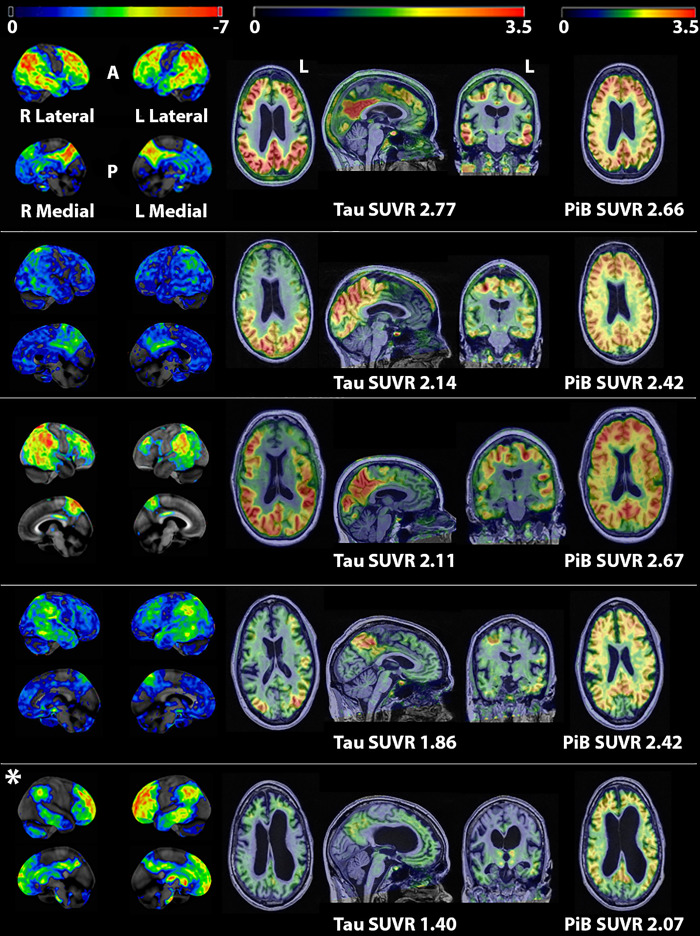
Discordance of CSF p-tau biomarkers and tau PET in five participants who had CSF p-tau in the normal range (< 61 pg/ml). *Left:* FDG–PET (using Cortex ID, GE Healthcare) with z-score bar at the top. *Middle:*^18^F-AV1451 tau PET with SUVR bar at the top and individual meta-ROI SUVR below each image. *Right:* PiB amyloid PET with SUVR bar at the top and individual meta-ROI SUVR below each image. Significant ^18^F-AV1451 uptake was present in all five participants (mean SUVR 2.05). CSF p-tau level, tau PET SUVR and mean time lag between CSF and tau PET for each participant can be found in Supplementary Table 1. *Bottom participant came to autopsy and was found to have A3, B3, C3 Alzheimer’s disease pathology.
